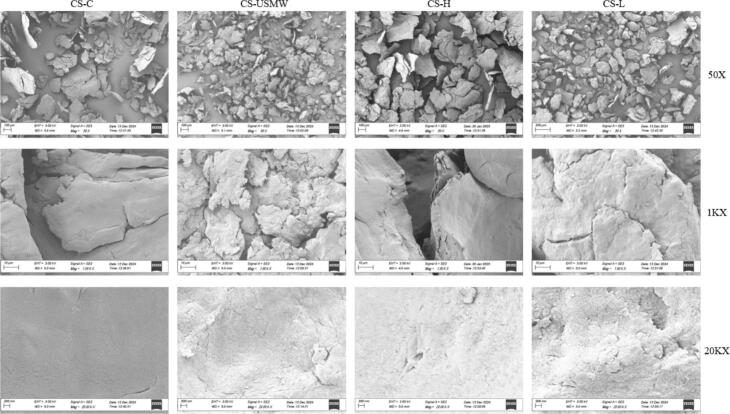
Surface morphology of chitosan analogue extractions by conventional extraction (CS-C), low-frequency ultrasound probe (CS-L), high-frequency ultrasound plate (CS-H), and ultrasound-microwave combination reactor (CS-USMW), at different magnifications (The treatments are labelled above the columns, and the magnifications are labelled at the end of each row).
